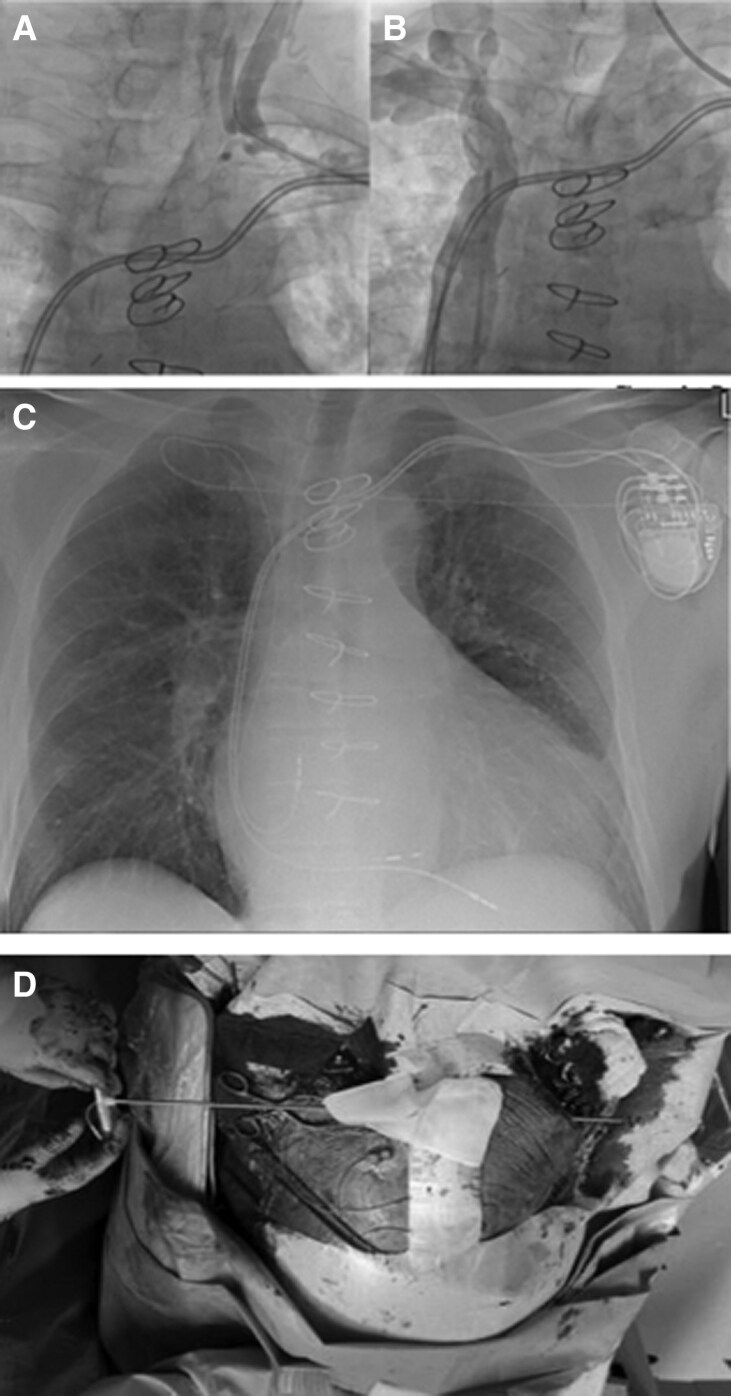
Upgrade of a dual-chamber pacemaker to a left bundle branch area pacing triple lead system in a patient with subclavian vein occlusion. (*A* and *B*) Venography from the left subclavian vein and the SVC by a sheath introduced from the femoral vein reveals a very long and complete total occlusion of the left subclavian vein. All attempts of reopening or passing the occlusion through collaterals were unsuccessful. (*C*)Thoracic X-ray after implantation of a left bundle branch pacing lead from the right subclavian vein. The lead is fixed with a sleeve on the right side, then tunnelled straight to the left-sided device pocket. (*D*) Operation situs. The tunnelling device (Codman disposable catheter passer), with a tapered nylon tip, has been pushed suprasternal to the pacemaker pocket on the left side. After the grab handle (white) has been removed, the IS1 connector tip is attached to the white inner plastic by a suture, and the olive tip with the plastic wire inside the tunnelling device can be pulled out towards the device pocket. Finally, the metal tube is removed towards the device pocket side.
